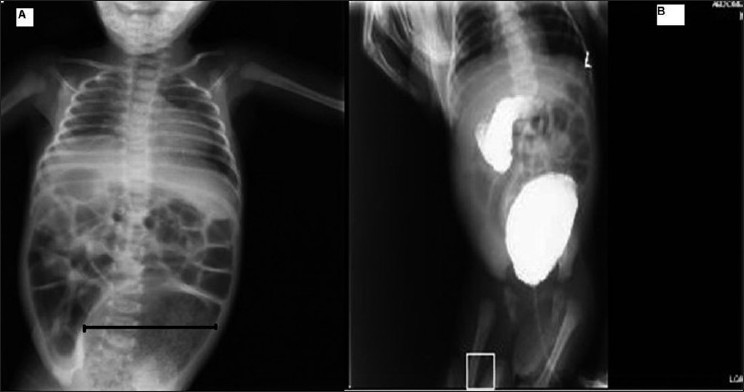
Plain X-ray showing dilated rectosigmoid, gas shadow occupying >50% of abdominal width. (b) Barium enema showing dilated rectosigmoid.
